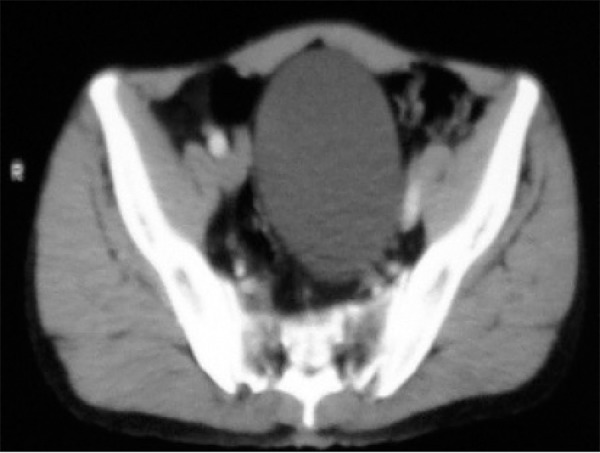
CT scan with contrast of the abdomen showing the median cystic image
